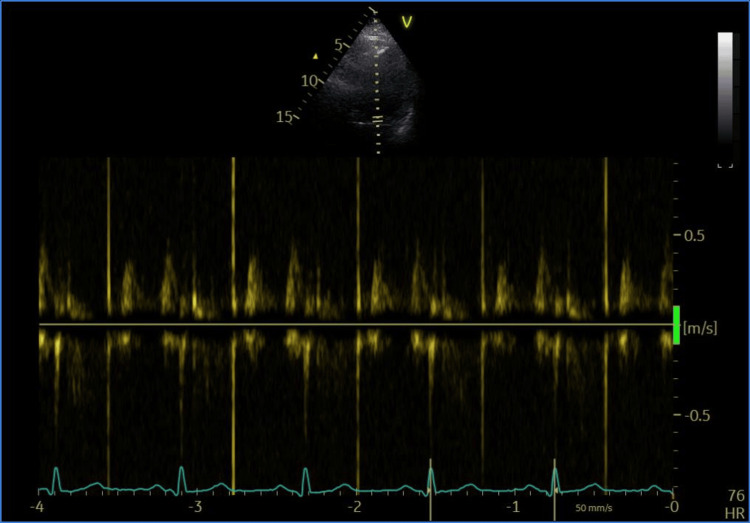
Post-operative transthoracic echocardiogram of the mitral valve.
